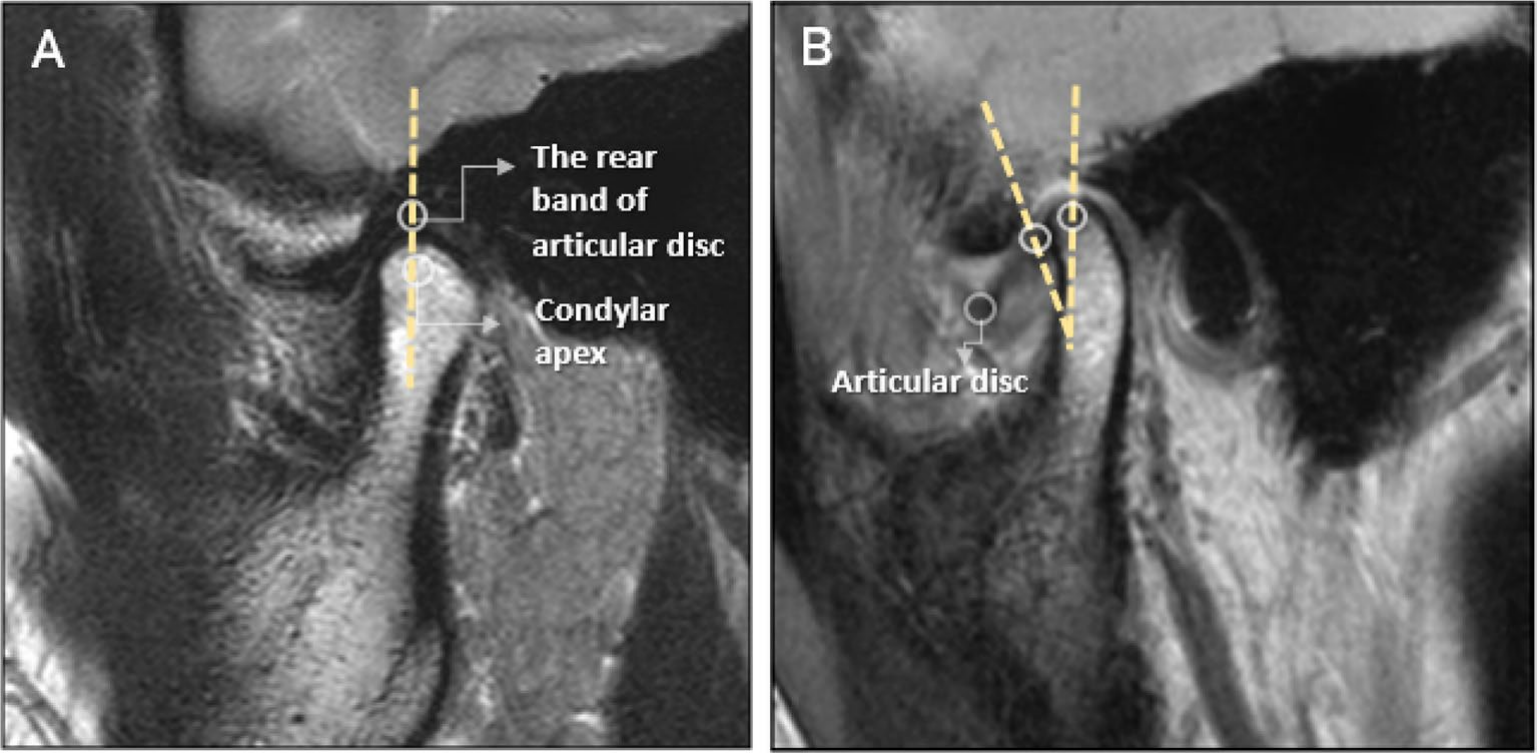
Magnetic resonance imaging (MRI) features of non-anterior disc displacement (non-ADD) (A) and ADD (B). Non-ADD: the rear band of the articular disc is located at 12 o’clock relative to the condylar apex at the closed position. ADD: the rear band of the articular disc moves forward beyond the normal range in the closed-mouth position.
